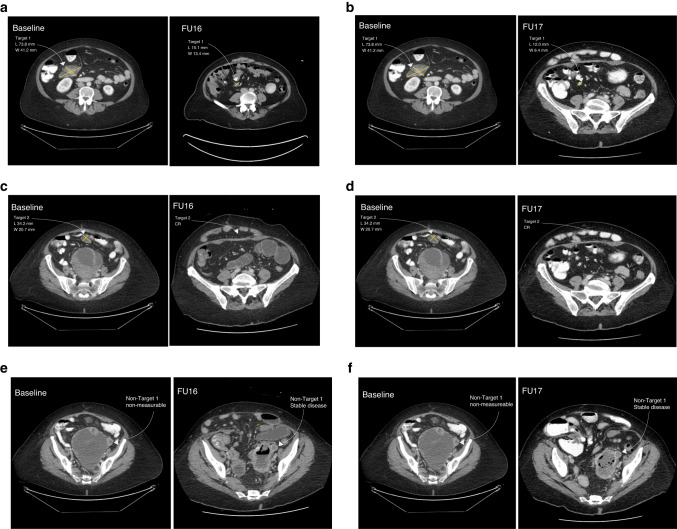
CT images showing changes from baseline in target and non-target lesions. CT images showing changes from baseline in target lesion 1 at FU 16 (a) and 17 (b), target lesion 2 at FU 16 (c) and 17 (d) and non-target lesion 1 at FU 16 (e) and 17 (f) in the patient with uterine leiomyosarcoma and experiencing a prolonged PR in cohort B1. CT computed tomography, FU follow-up, PR partial response. NB. Baseline = 20 Dec 2017; FU 16 = 14 Jan 2020; FU 17 = 27 Apr 2020.
